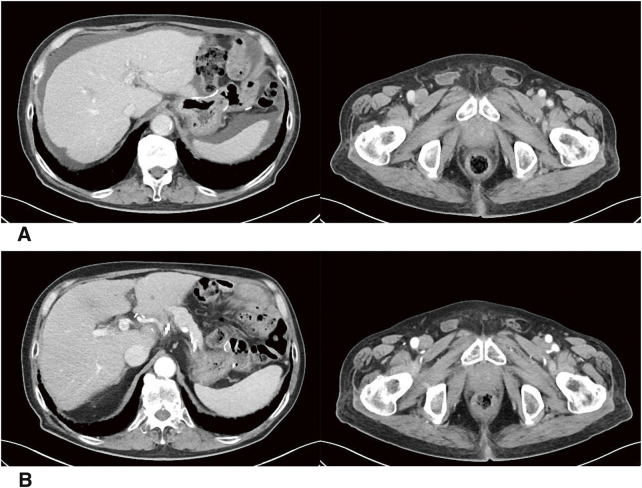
Postoperative CT findings. Four months after surgery, no evident peritoneal dissemination nodules were observed; however, ascites were detected, raising suspicion of recurrent peritoneal dissemination (A). Nivolumab monotherapy was resumed, and the ascites gradually decreased. The patient has continued to show PR on CT scans after 17 courses of nivolumab (B). CT, computed tomography; PR, partial response
